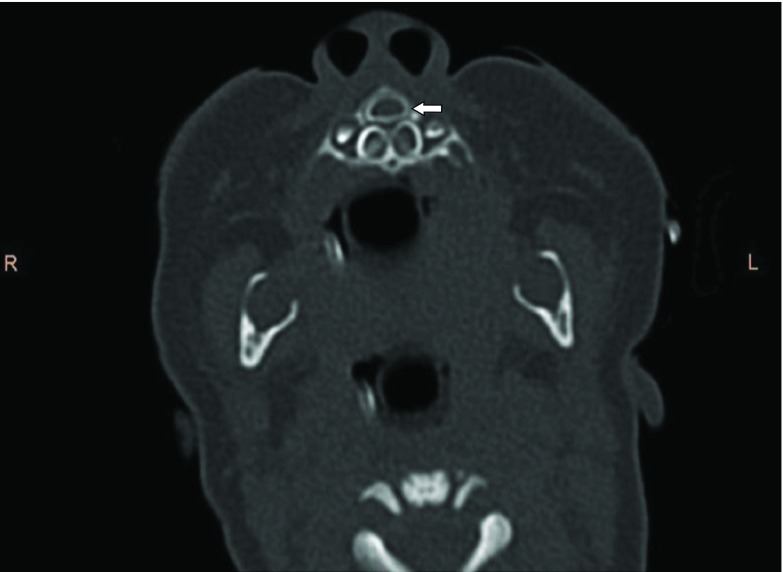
CT image (pre-stenting): axial view of the central mega-incisor (white arrow).
